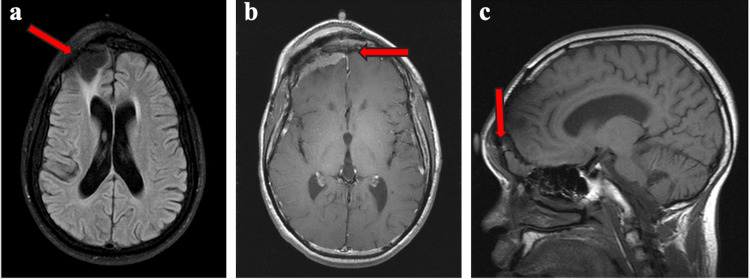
Brain MRI with and without contrast (August 2022). The red arrows indicate key imaging findings: (a) an axial T2 FLAIR image demonstrating diffuse cerebral volume loss with postsurgical right frontal encephalomalacia; (b) an axial post-contrast T1-weighted image showing a thick, irregular right frontal extra-axial enhancing rind consistent with recurrent tumor; and (c) a 0.9-cm extra-axial enhancing lesion adjacent to the right frontal lobe extending through the craniotomy defect toward the superior orbit.
